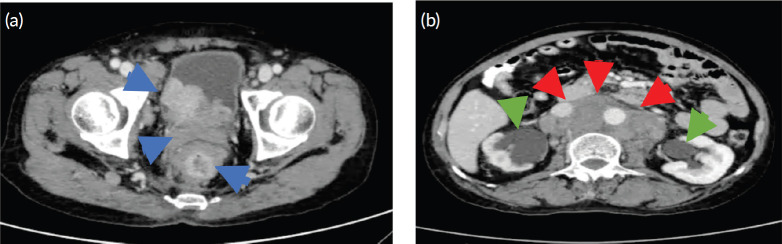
Representative axial cut images of the abdominopelvic CT scan with oral, rectal and intravenous contrast. (a):. The prostate gland is enlarged and converted to a heterogenous mass 6.8 × 5.2 × 5.4 cm in size and a volume of 99.3 cc with intravesical extension and cannot be separated from a rectal mass (blue arrows). (b): Confluent, matted, markedly enlarged retroperitoneal lymph nodes (red arrows) and dilated urinary collecting systems and ureters (green arrows).
